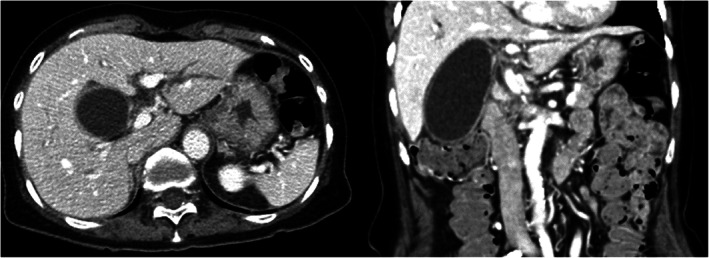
Abdominal computed tomography shows remarkable swelling of the gallbladder, thickness of the gallbladder wall and exclusion of the common hepatic duct, although there was no evidence of malignant tumors
